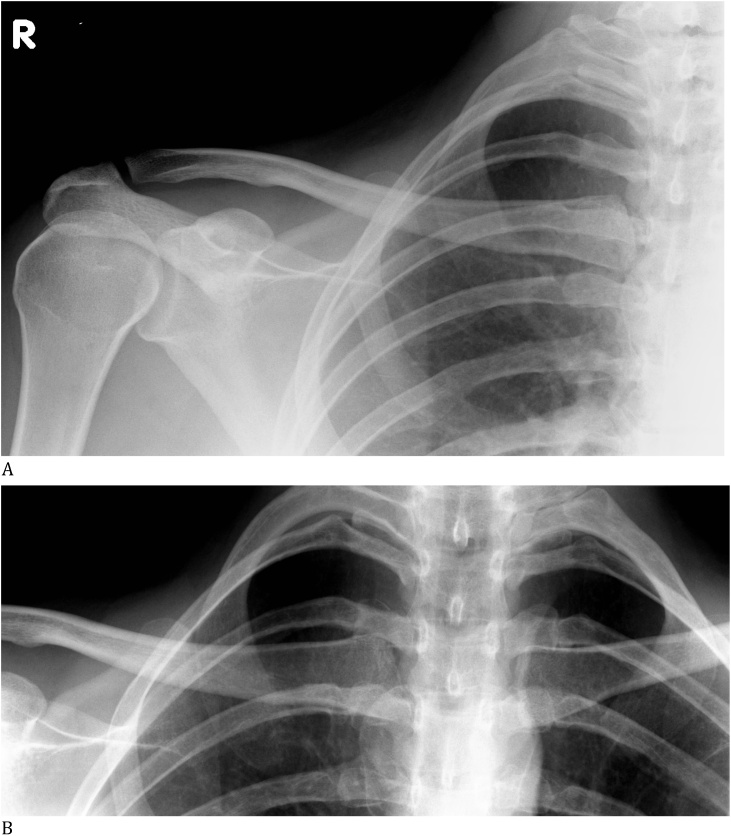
A: AP radiograph of right clavicle on presentation which showed no obvious fracture or dislocation of the shoulder girdle. B: AP radiograph of bilateral sternoclavicular joints showed subtle joint space widening on the right side. The overlapping of medial structures such as the ribs, clavicle, sternum and vertebrae impedes visualisation of the sternoclavicular joint in direct antero-posterior films.
